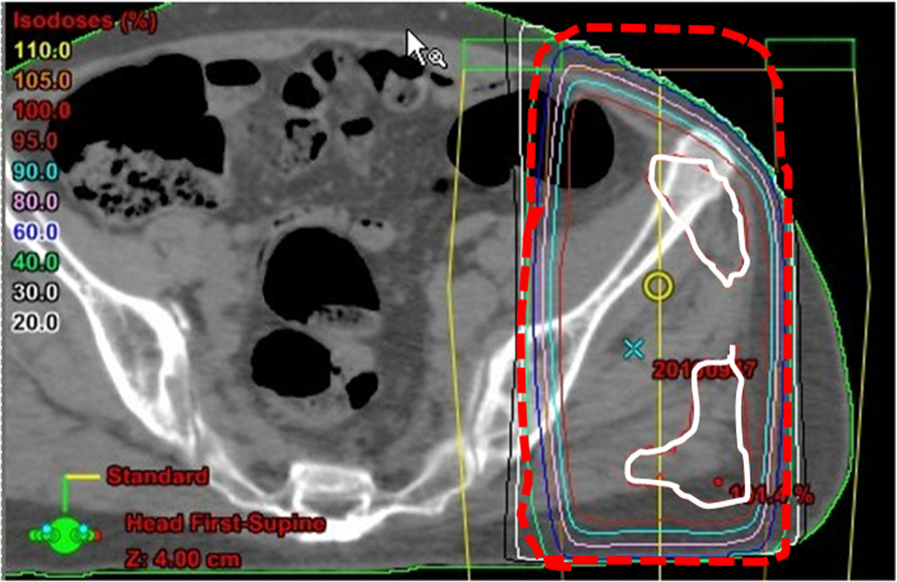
Computed tomography revealed the irradiated area; the white area received 100%, and the dotted-area received 60% of the irradiation.
